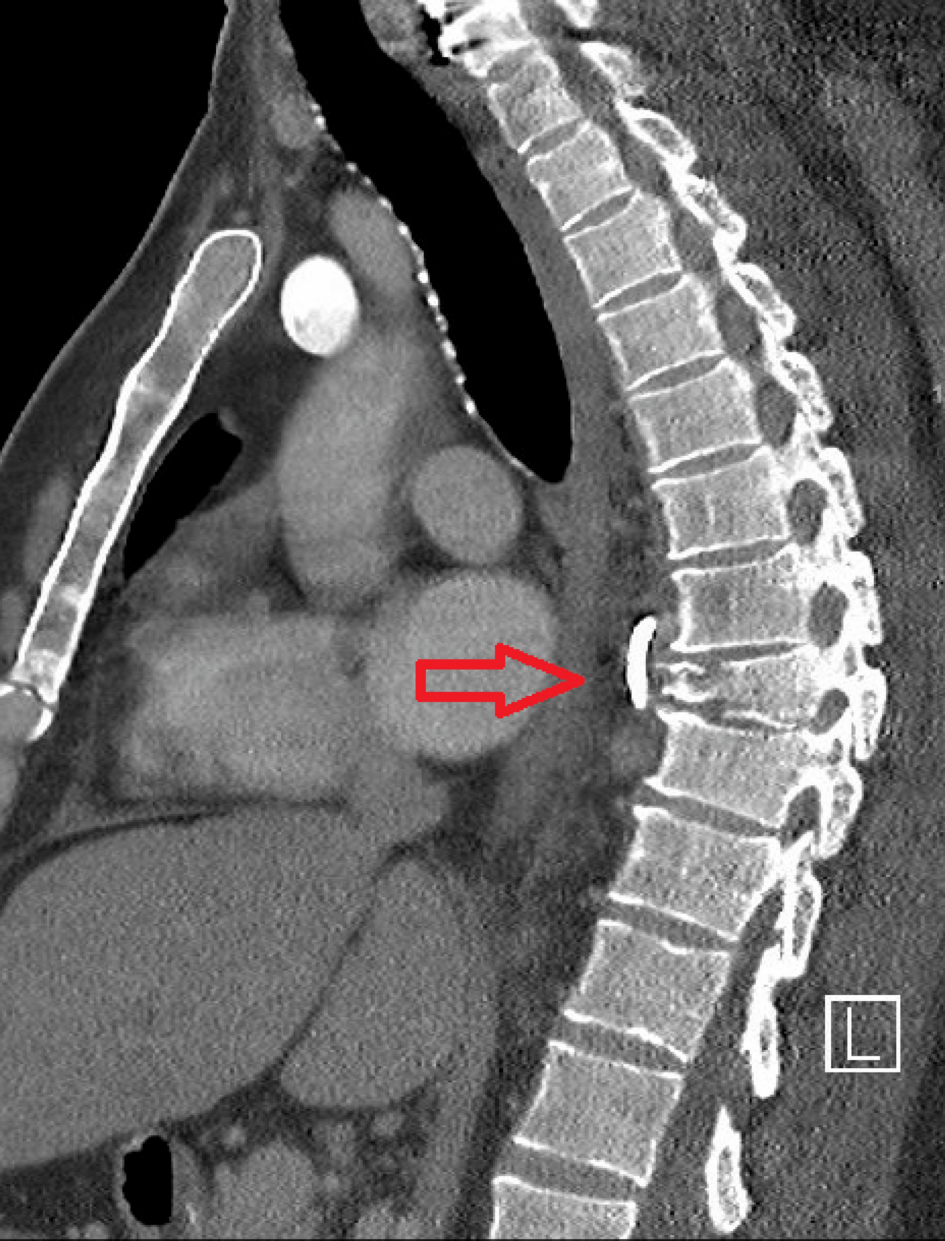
CT imaging showing paravertebral fluid collection around the T8 fracture.
